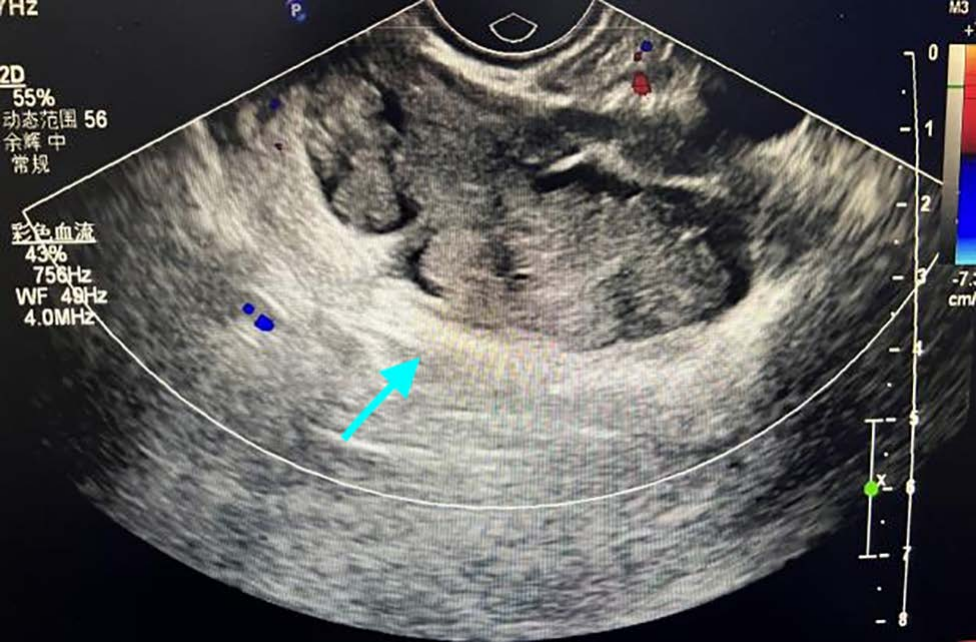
Vaginal ultrasound showed a mixed echogenic mass behind the rectum, and the echogenicity showed dough sign and short line sign without blood flow signal, considering mature teratoma.
